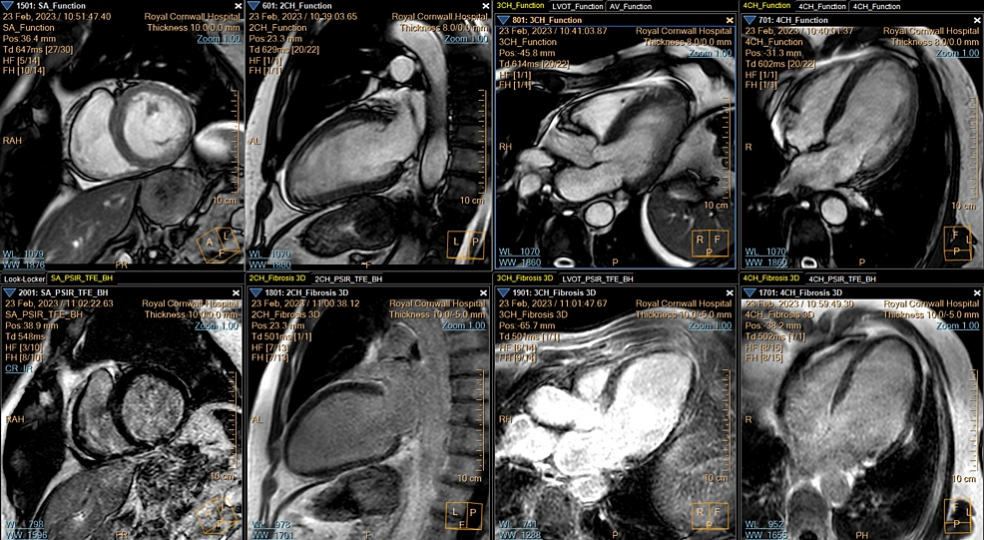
Cardiac MRI images showing viable segments Left to right (top and bottom): cross-section, anterior wall, anteroseptal wall, and septum MRI: magnetic resonance imaging
